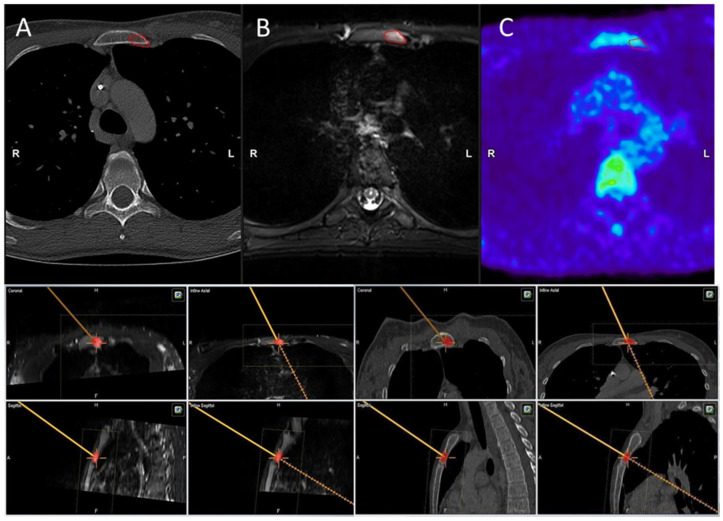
A 35-year-old patient with a solitary metastasis in the manubrium sterni with breast carcinoma. The tumor was not visible in the CT scan (A), only in MRI (B) and PET-CT (C).
